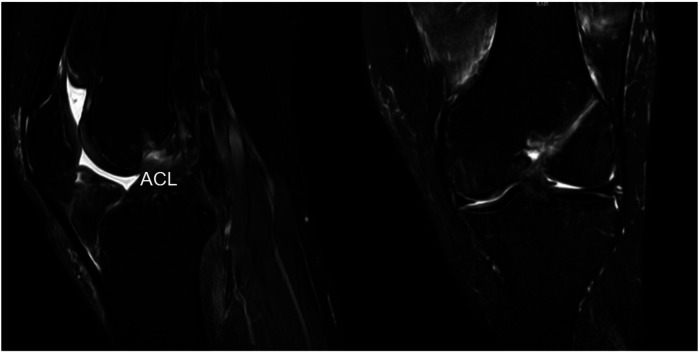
MRI scans of the sagittal and coronal planes showing good morphology after fixation.
